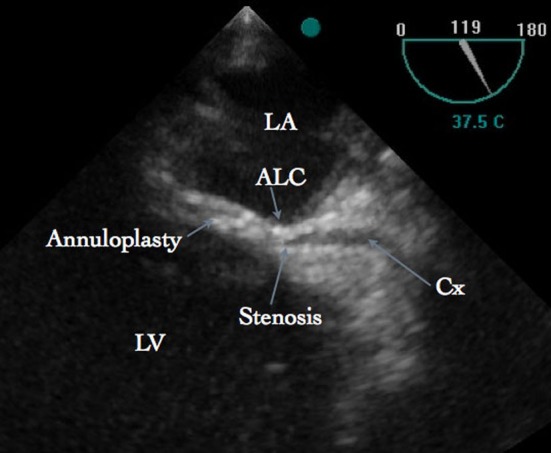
Transesophageal echocardiography showing stenosis of the circumflex artery. ALC, Anterolateral commissure; Cx, Circumflex artery; LA, Left Atrium; LV, Left Ventricle.
